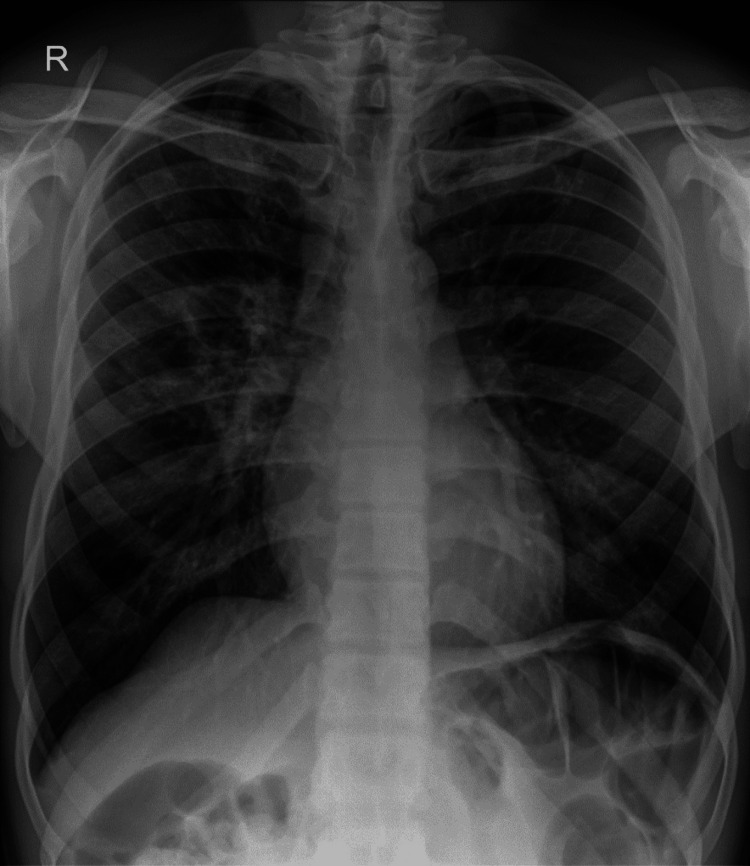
Example of a PA chest X-ray image with de-identified patient data. PA: posteroanterior
